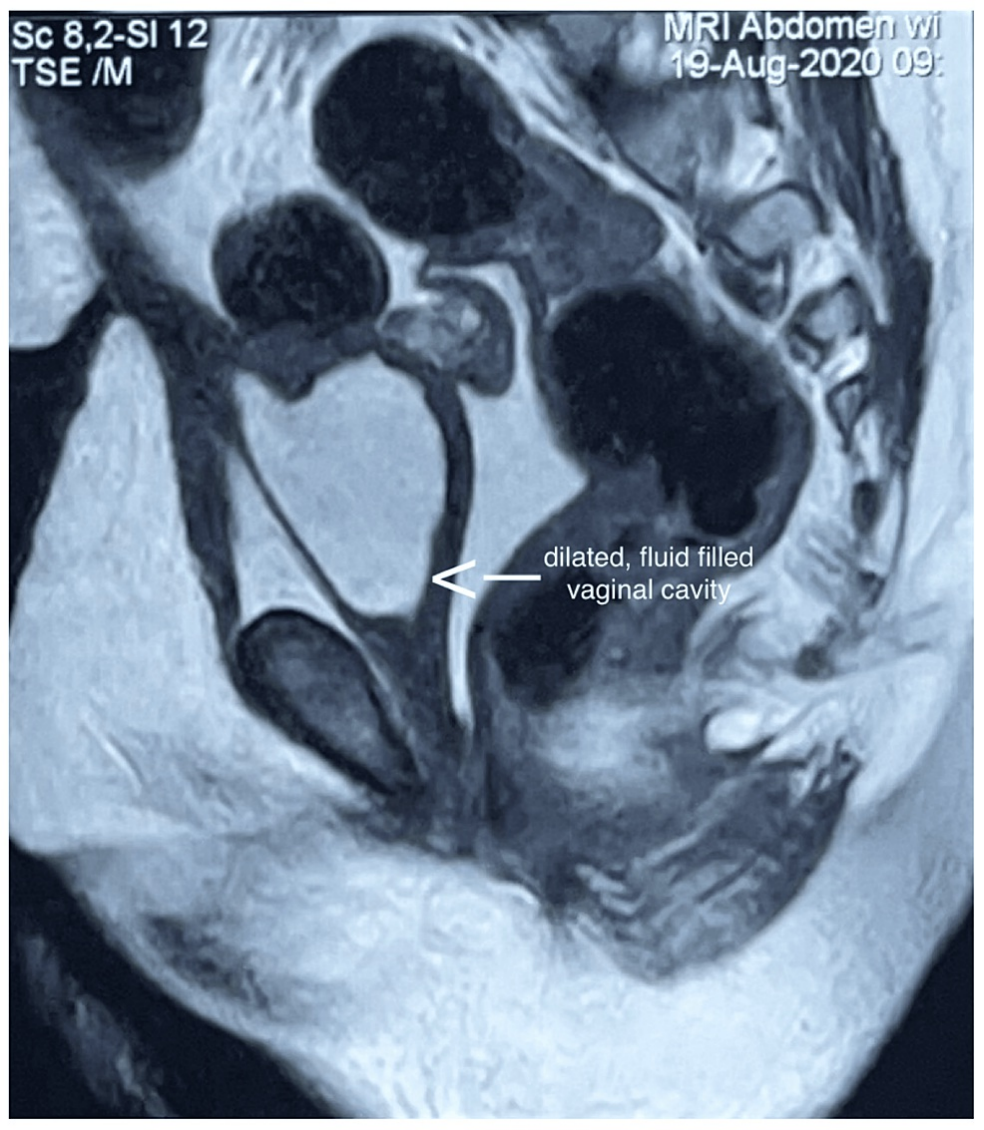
Magnetic resonance imaging scan of the abdomen and pelvis done at four years of age showing a dilated, fluid-filled vaginal cavity.
